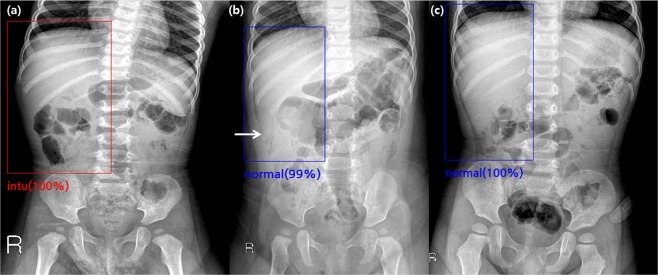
False-positive and false-negative cases found by the algorithm in the validation set. (a) A supine abdominal radiograph of a 15-month-old boy without intussusception. One radiologist and the algorithm reported false-positive results, while the other three radiologists reported true-negative results. (b) A supine abdominal radiograph of a 10-month-old boy with intussusception. One radiologist and the algorithm reported false-negative results, while the other three radiologists reported true-positive results. (c) A supine abdominal radiograph of a 29-month-old boy with intussusception. All of the four radiologists and the algorithm reported false-negative results.
